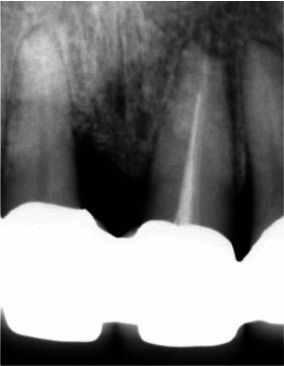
Peri-apical radiograph at 2 years post-surgery, with a substantial stability of the grafted bone, and significant adaptation of the prosthetic elements
